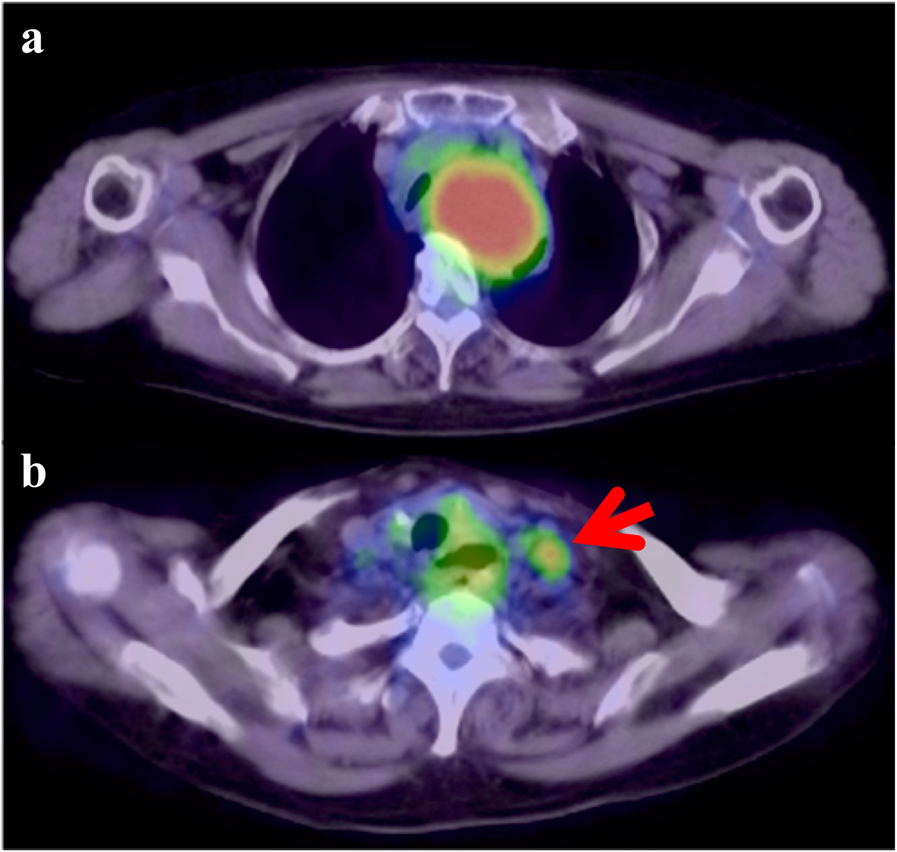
^18^F-fluorodeoxyglucose (FDG)-positron emission tomography image revealed an upper mediastinal homogeneous mass (a) and left supraclavicular lymph node with increased FDG accumulation (b)
